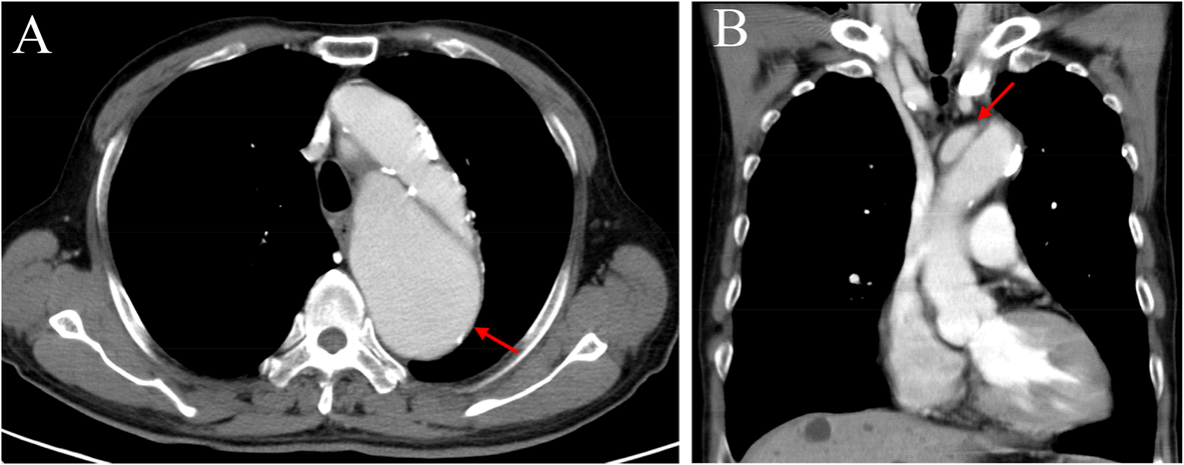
Axial (a) and coronal (b) view in contrast-enhanced computed tomography revealed dissecting flap involving the ascending and descending aorta (red arrow), and diagnosed with type A aortic dissection
